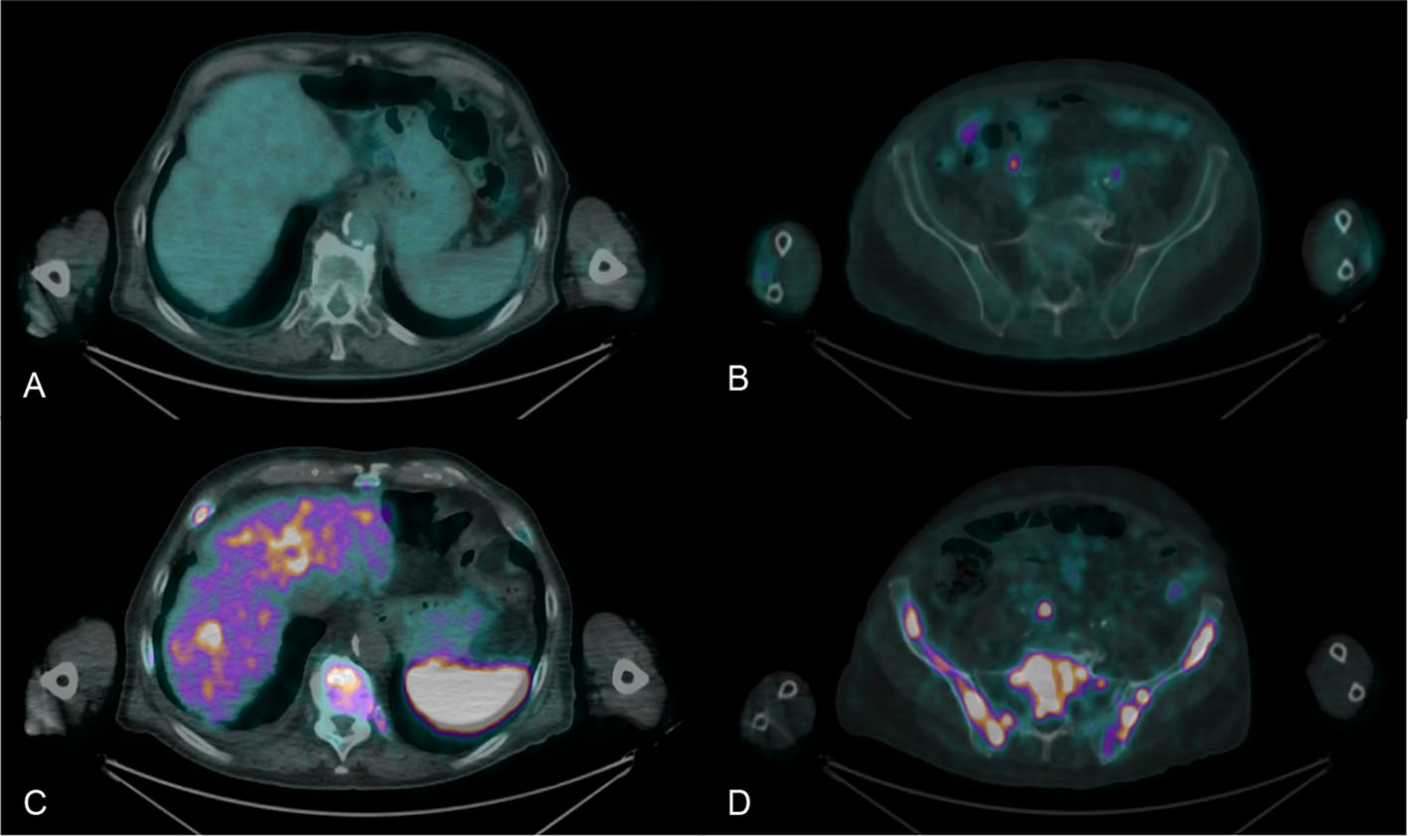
[^18^F]FDG PET/CT showed no significant uptake both in the liver parenchyma (a) or skeletal district (b). In contrast, [^68^Ga]Ga-DOTA-TOC PET/CT revealed pathologically inhomogeneous uptake in the liver parenchyma (c) and multiple lesions with increased uptake in the pelvic bones (d)
